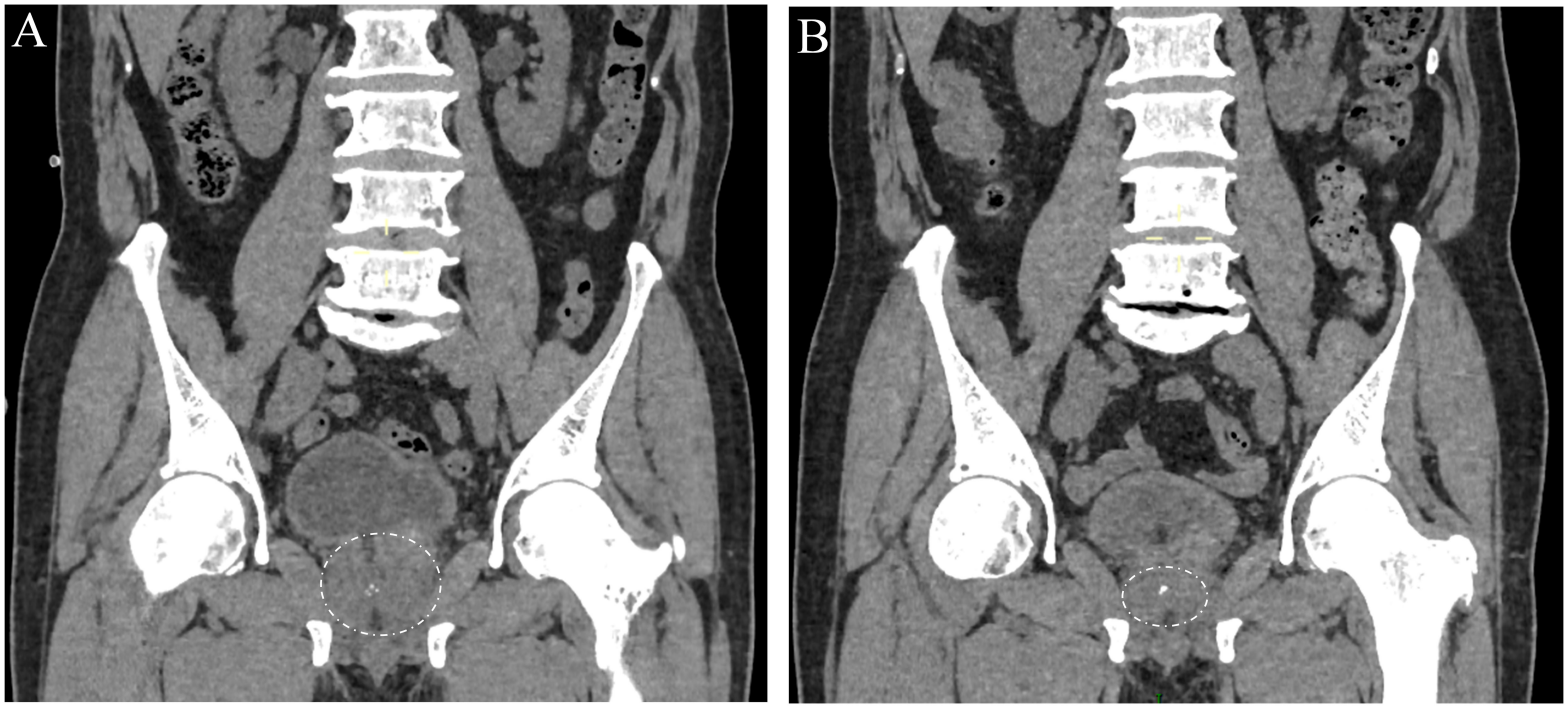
Computed tomography (CT) imaging of the pelvis without intravenous contrast at the level of the prostate during diagnosis and treatment stages. (A) Prior to treatment initiation. (B) Following completion of six cycles of chemotherapy combined with androgen receptor pathway inhibition. White circles indicate the boundary of the primary prostatic tumor, demonstrating substantial regression after therapy.
